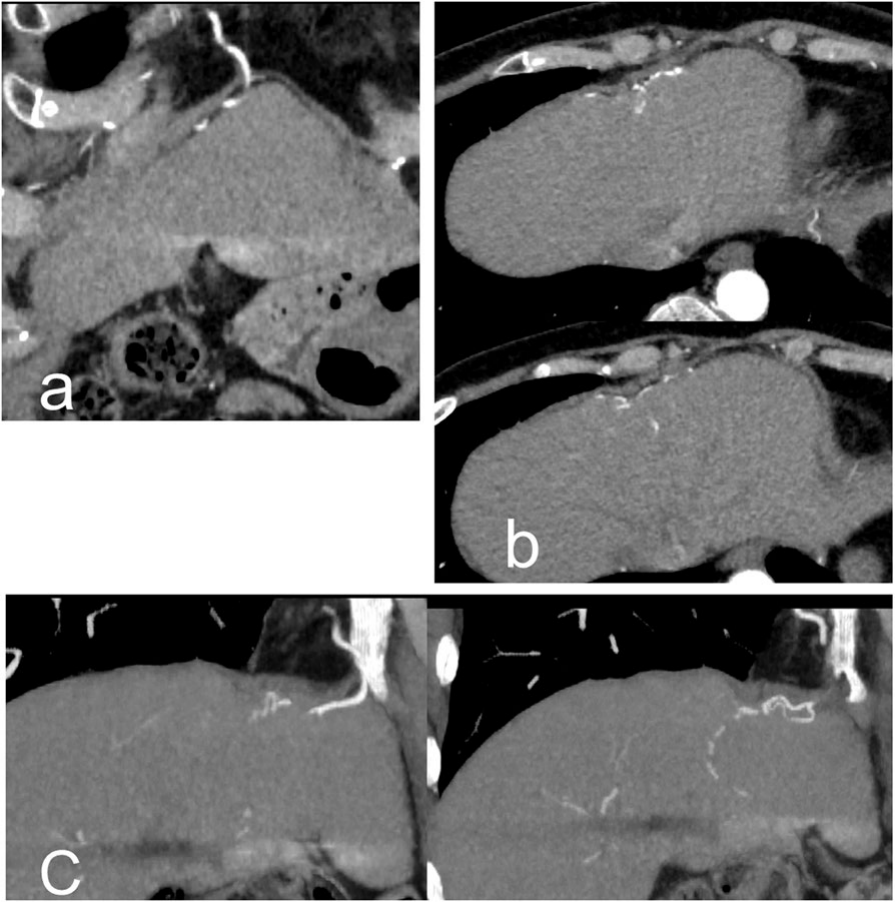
The right internal thoracic artery was connected to the intrahepatic artery through Sappey’s superior artery on the coronal (a), axial (b), and oblique (c) post-ET CTA images in case 5
